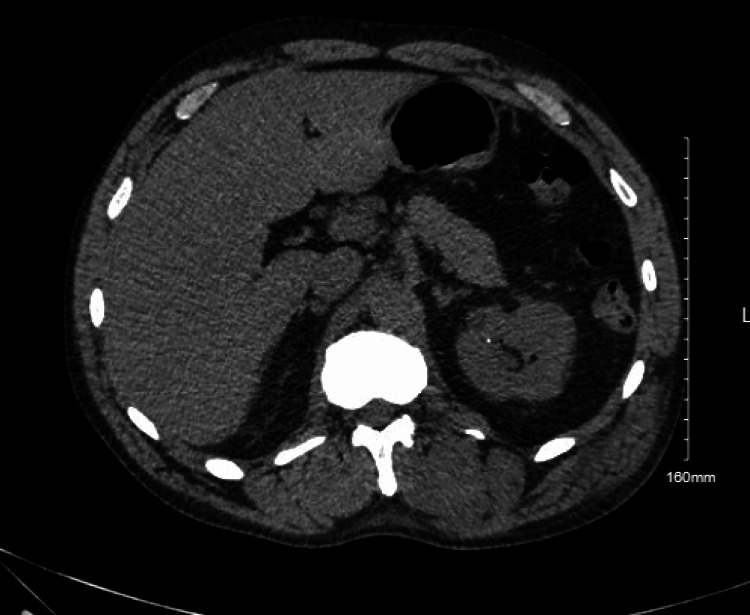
CT abdomen and pelvis demonstrating atrophy and cortical scarring involving the left kidney.
